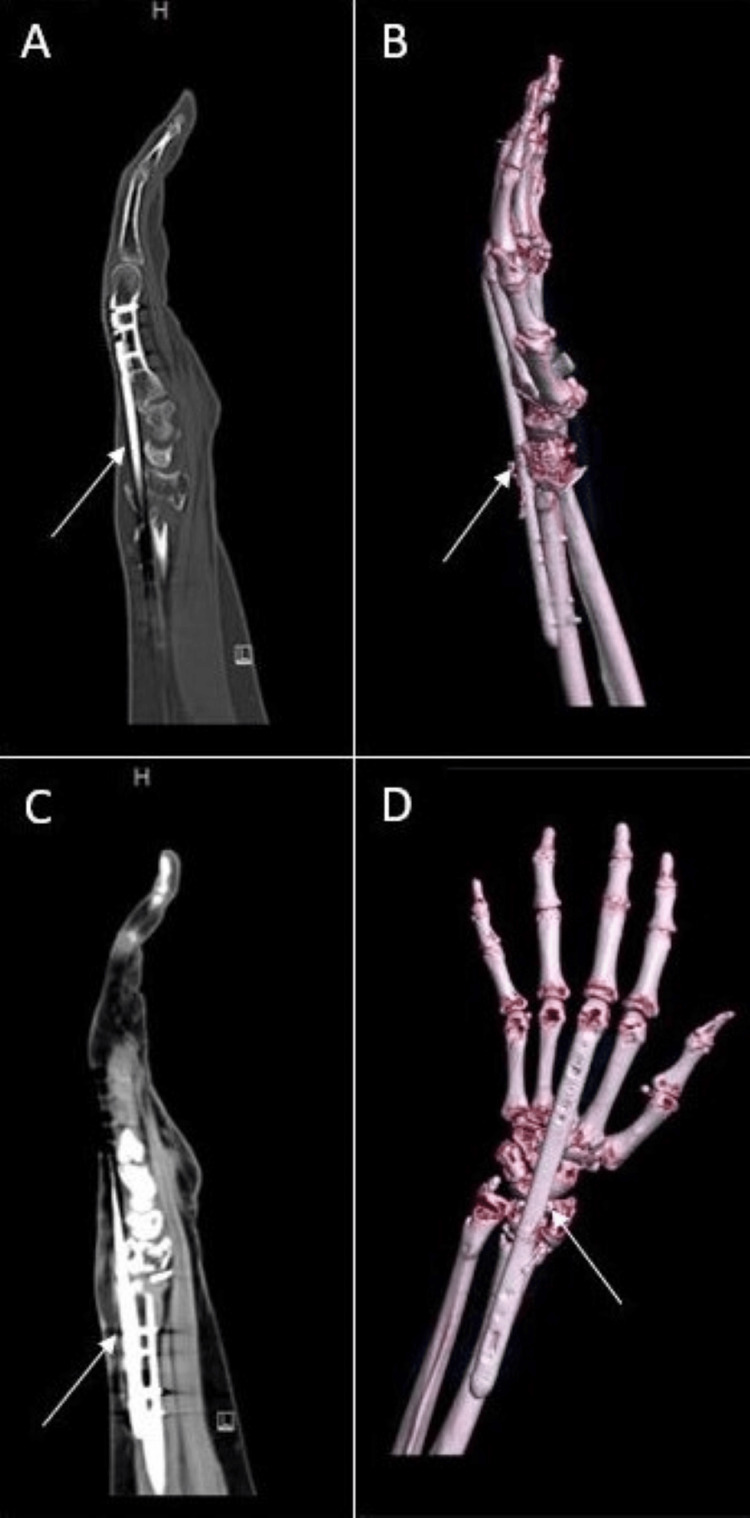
Initial fixation: three-month postoperative films A/C: CT demonstrating the DSP in satisfactory position with fracture healing, but malalignment overall B/D: 3D CT reconstruction films
